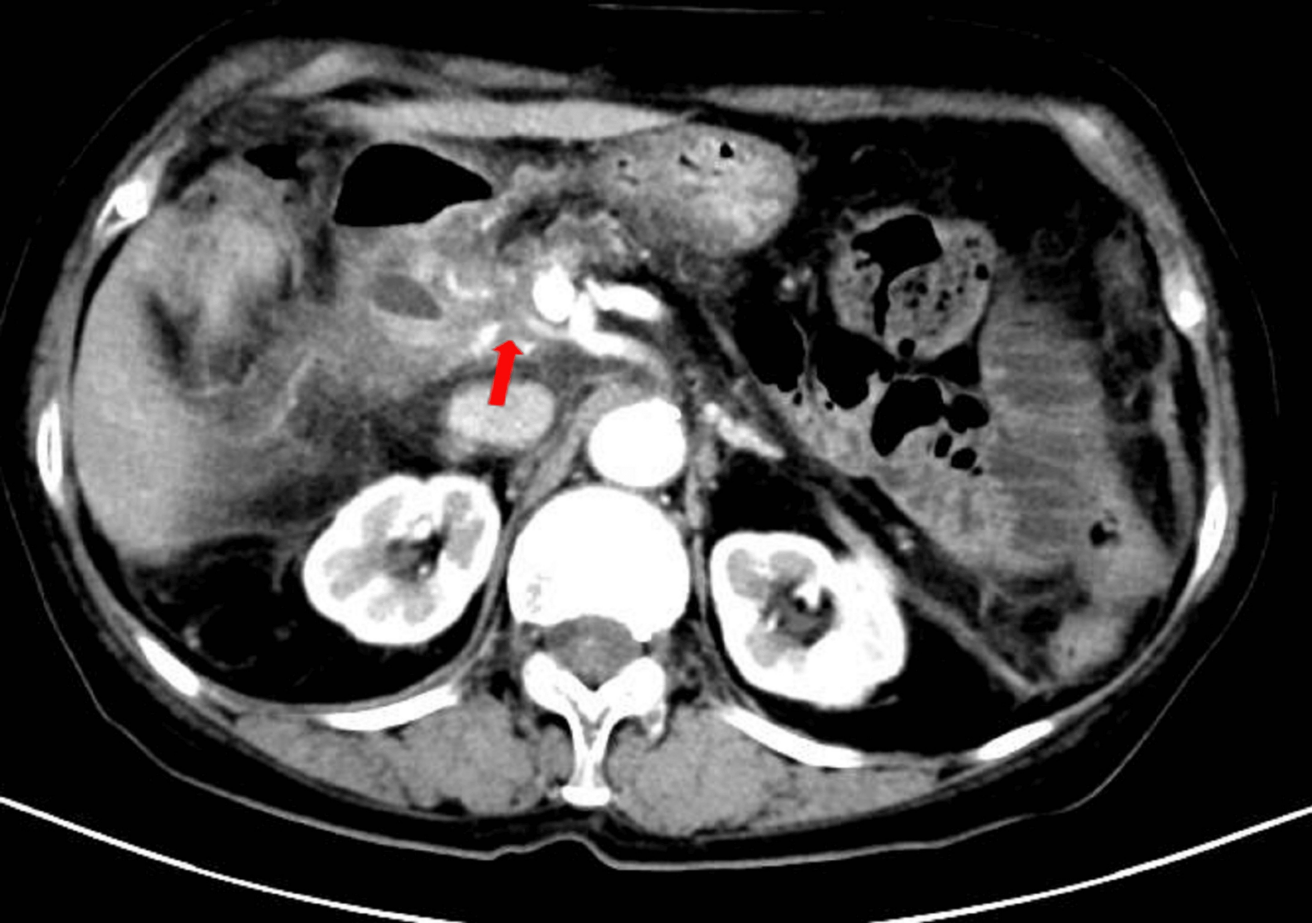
Enhanced CT on August 26, 2023, showing more significant oozing next to the pancreatic head mass and increased pelvic fluid, with no significant changes elsewhere. The tumor response has been assessed as SD (red arrow) CT: computed tomography; SD: stable disease
